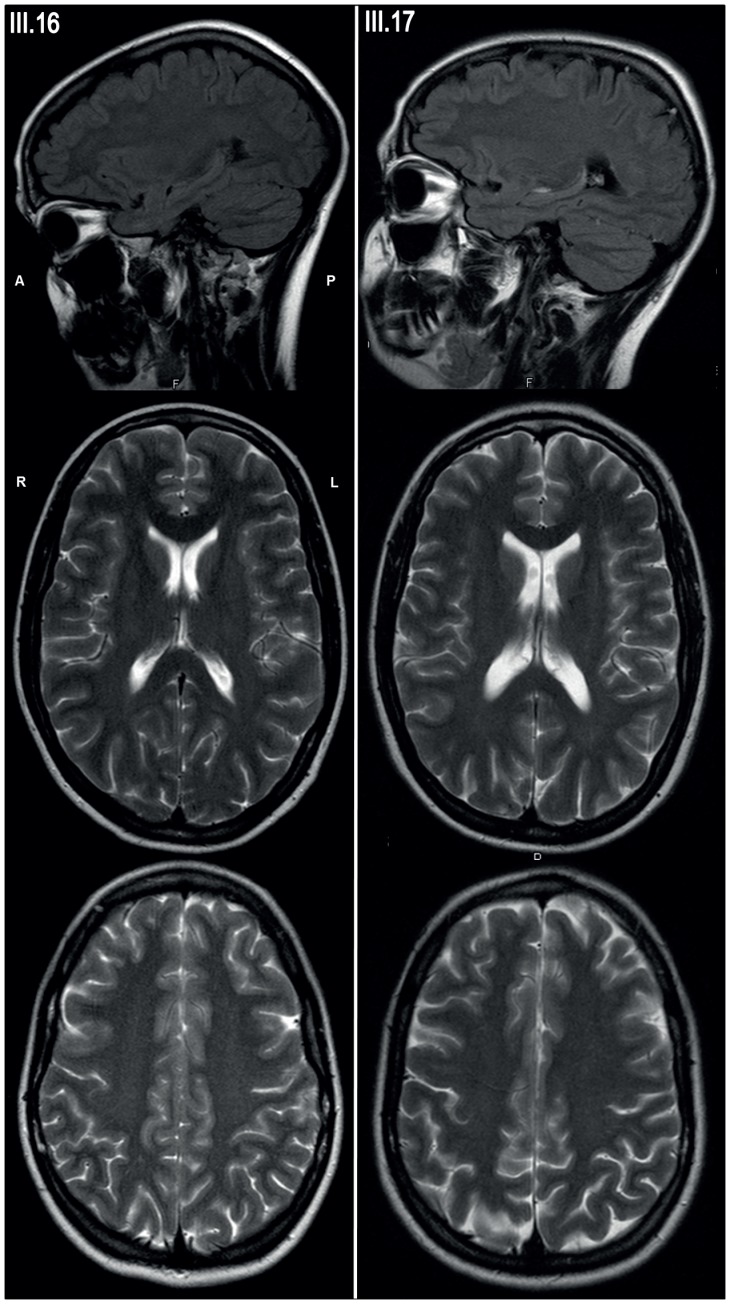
MR images of family members that were negative for D313Y did not show any white matter changes. Upper row: Sagittal FLAIR-sequence; median and lower row: Axial T2-weighted sequences of subject III.16 and III.17.
